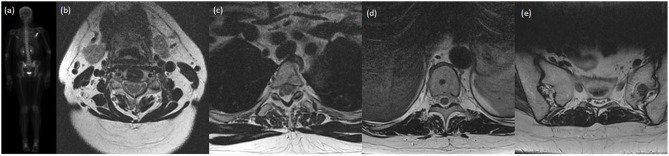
A case presentation of a lady with invasive ductal carcinoma of the breast who was treated definitively with lumpectomy, adjuvant chemotherapy, and adjuvant radiotherapy. Shortly after completion of therapy, on re-staging investigations, she was found to have oligometastatic disease in the bones, specifically at C4, T3, T10, the left sacral ala, and right scapula. She received SBRT to each site and was started on hormonal therapy. At most recent follow-up 20 months later, she has not had progression of known disease nor interval development on new metastatic disease. (a) Posterior-anterior projection of pre-treatment bone scan demonstrating increased uptake within the right scapula, T3 and the right sacral ala. Subsequent images of axial slice of T2-weighted MRI demonstrating near complete marrow replacement of C4 (b), focal marrow abnormality in posterior T3 body (c), rounded focus centrally of the T10 vertebral body (d), and 13 mm lesion of left sacral ala (e).
